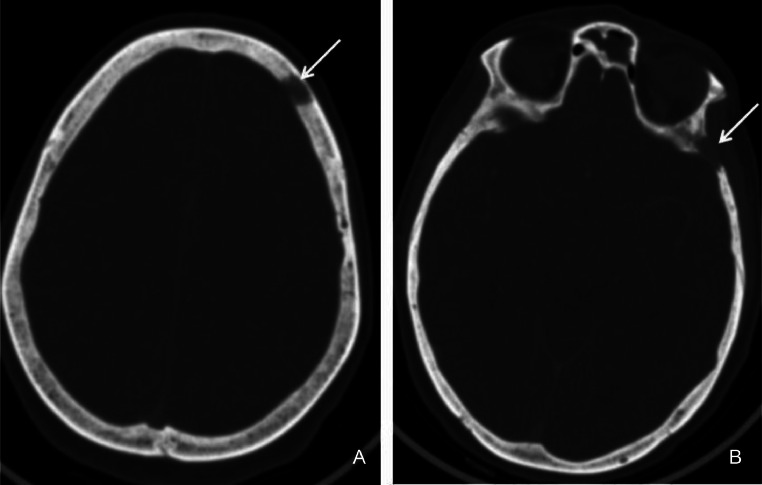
Axial section of brain CT scan showing left frontal (A) and temporal (B) osteolytic lesions.
